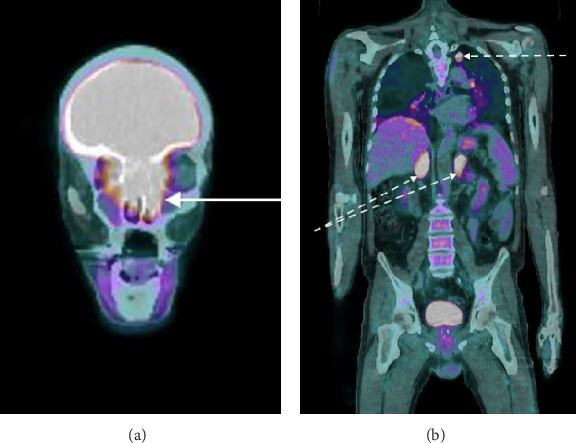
^18^FDG-PET scan at diagnosis showing an intensely hypermetabolic lesion (SUVmax of 8, compared to a hepatic reference of 2) corresponding to a tumoral infiltration originating from the ethmoid, extending into the nasal cavities, without evidence of cervical lymphadenopathy (a, solid white arrow). A nodular left apical pulmonary nodule (SUVmax of 7) is also observed, along with bilateral adrenal hyperplasia (SUVmax of 8) consistent with ACTH-dependent hyperstimulation (b, dotted white arrows).
